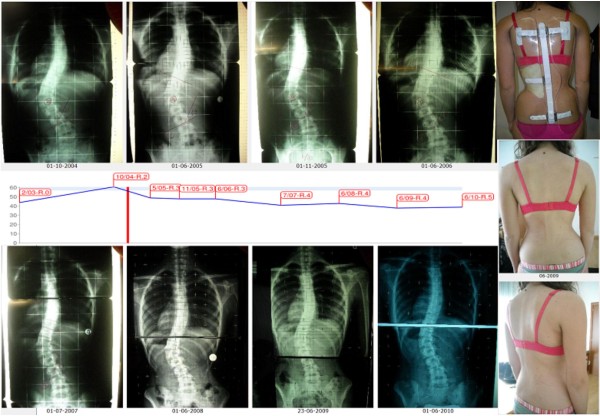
First case report: adolescent thoraco-lumbar scoliosis patient over 45° who reached the end of treatment. On the left, from left to right, in the first and then in the third line: all x-rays of this case report of a patient that reached the end of treatment (each x-ray is marked with the corresponding date). On the left, midline: graph with the results obtained, dates and Risser test. On the right, in top-down direction: the brace used, and the posterior and sagittal aesthetic appearance at the end of treatment. A.A. has been evaluated the first time in December 2004, presenting with a second x-ray showing a thoraco-lumbar left scoliosis progressed in 18 months from 44° (Risser 0) to 61° (Risser 2). Fusion had been proposed, but refused. She started treatment with the Sforzesco brace 23 hours per day and SEAS exercises 3 times a week (45'): after 5 months she was 49° (Risser 3). Treatment continued other 6 months 23 hours per day, then 22 per 6 months, and brace was continously and gradually weaned 2 hours every 6 months: she improved after 3 years of treatment (41°, Risser 4) and 4 years (38°, Risser 4). At the last x-ray after 48 hours without the brace, and 5 years and 6 months of continuos Sforzesco brace treatment and SEAS exercises, she finished treatment at 39°.
